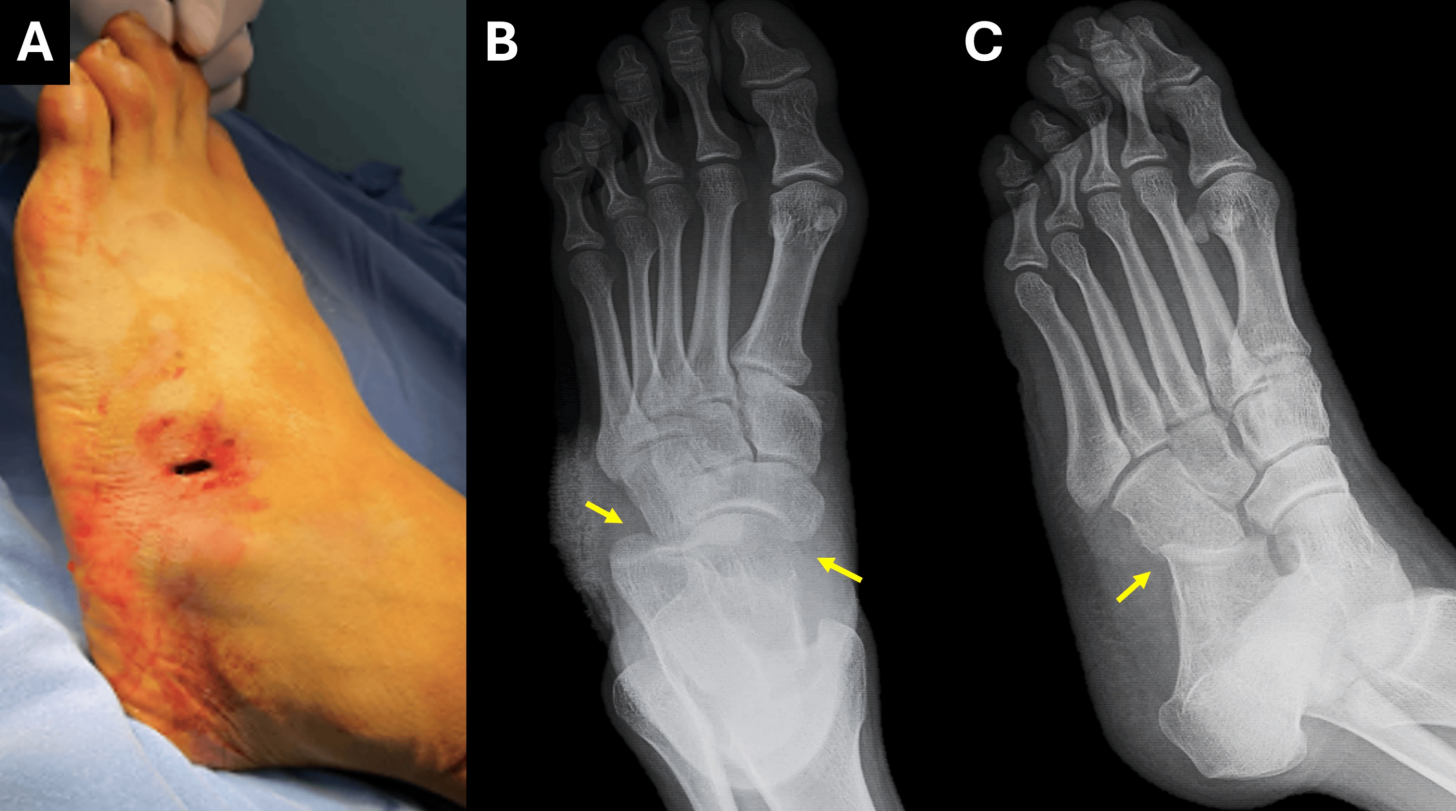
Clinical and radiographic findings of isolated calcaneocuboid dislocation. (A) Intra-operative clinical image of the left foot showing a 1 cm dorsal laceration over the lateral midfoot, located at the level of the fifth metatarsal, with clean margins and no visible contamination, consistent with high-energy traumatic injury. (B) Pre-operative anteroposterior radiograph demonstrating lateral displacement and articular incongruity at the calcaneocuboid joint (yellow arrows), without evidence of associated fractures. (C) Oblique view confirming isolated dislocation of the calcaneocuboid joint, with preserved alignment of adjacent joints and no radiographic signs of bony fragmentation, supporting the diagnosis and facilitating surgical planning (yellow arrow).
